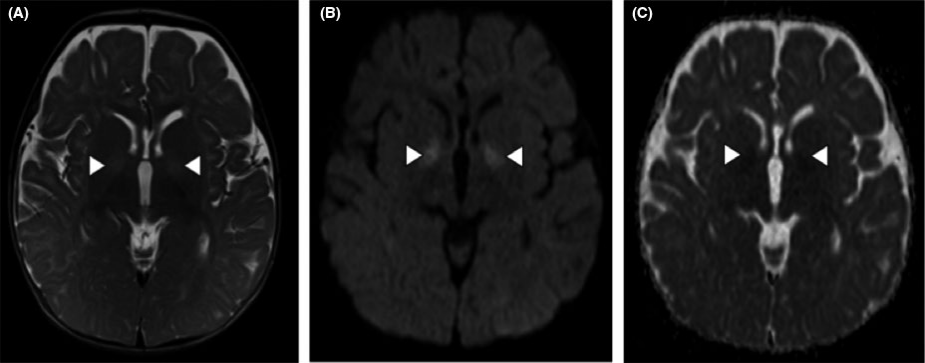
MR imaging of the brain demonstrating bilateral symmetric high signal intensity in the globi pallidi (white arrowheads) on T2‐weighted images (A), with corresponding high signal intensity of diffusion‐weighted images (DWI) (B), and low signal intensity on apparent diffusion coefficient (ADC) maps (C), consistent with diffusion restriction
